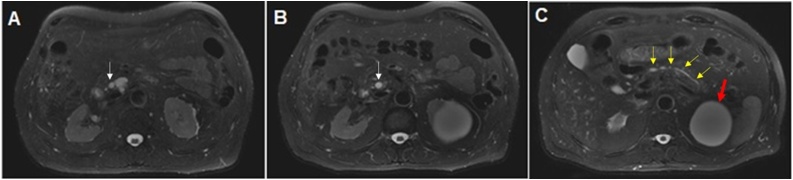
Axial fat-suppressed T2-weighted MRI sequences. A: Presence of a BD-IPMN located in the head of the pancreas measuring 22 × 17 mm (white arrow) B: MD-IPMN measuring 10.4 mm extending from the head to the neck of the gland (white arrow). C: Normal main pancreatic duct in the pancreatic body and tail (yellow arrows) and large left renal cyst classified as Bosniak 1 (red arrow).gr1
